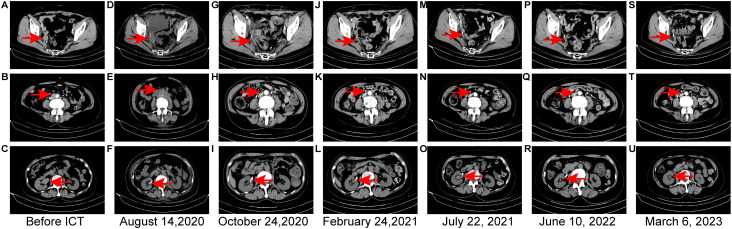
Computed tomography (CT) images during second-line therapy. (A–C) CT images before second-line therapy. (A) Enlarged lymph node adjacent to the right pelvic wall, significant enhancement following contrast administration performed on May 18, 2020. (B) Enlarged lymph node in the retroperitoneal area, significant enhancement following contrast administration performed on May 18, 2020. (C) Distended right renal pelvis and ureter on July 18, 2020. (D–F) CT on August 14, 2020 after two cycles of immunochemotherapy. (D) Enlarged lymph node adjacent to the right pelvic wall. (E) Enlarged lymph node in the retroperitoneal area. (F) No evidence of dilatation in the right renal pelvis or ureter following the insertion of the J-tube. (G–I) CT images on October 24, 2020 after completing four cycles of immunochemotherapy. (G) Metastatic lymph nodes adjacent to the right pelvic wall significantly decreased in size. (H) Metastatic lymph nodes in the retroperitoneal region significantly decreased in size. (I) No evidence of dilatation in the right renal pelvis or ureter. (J–L) CT images on February 24, 2021 after completing five cycles of immunochemotherapy. (M) Metastatic lymph nodes adjacent to the right pelvic wall significantly decreased in size. (N) Metastatic lymph nodes in the retroperitoneal region significantly decreased in size. (O) No evidence of dilatation in the right renal pelvis or ureter. (M–O) CT images on July 22, 2021 after completing six cycles of immunochemotherapy showed that the metastatic lymph nodes in the retroperitoneal region and adjacent to the right pelvic wall had reduced in size relative to prior imaging. Furthermore, medial displacement of the right ureter was noted. (P–R) CT scan conducted on June 10, 2022–2 years following the diagnosis of the second recurrence showed that multiple small lymph nodes in the retroperitoneal region and adjacent to the right pelvic wall. (S–U) CT scan conducted on March 6, 2023.
